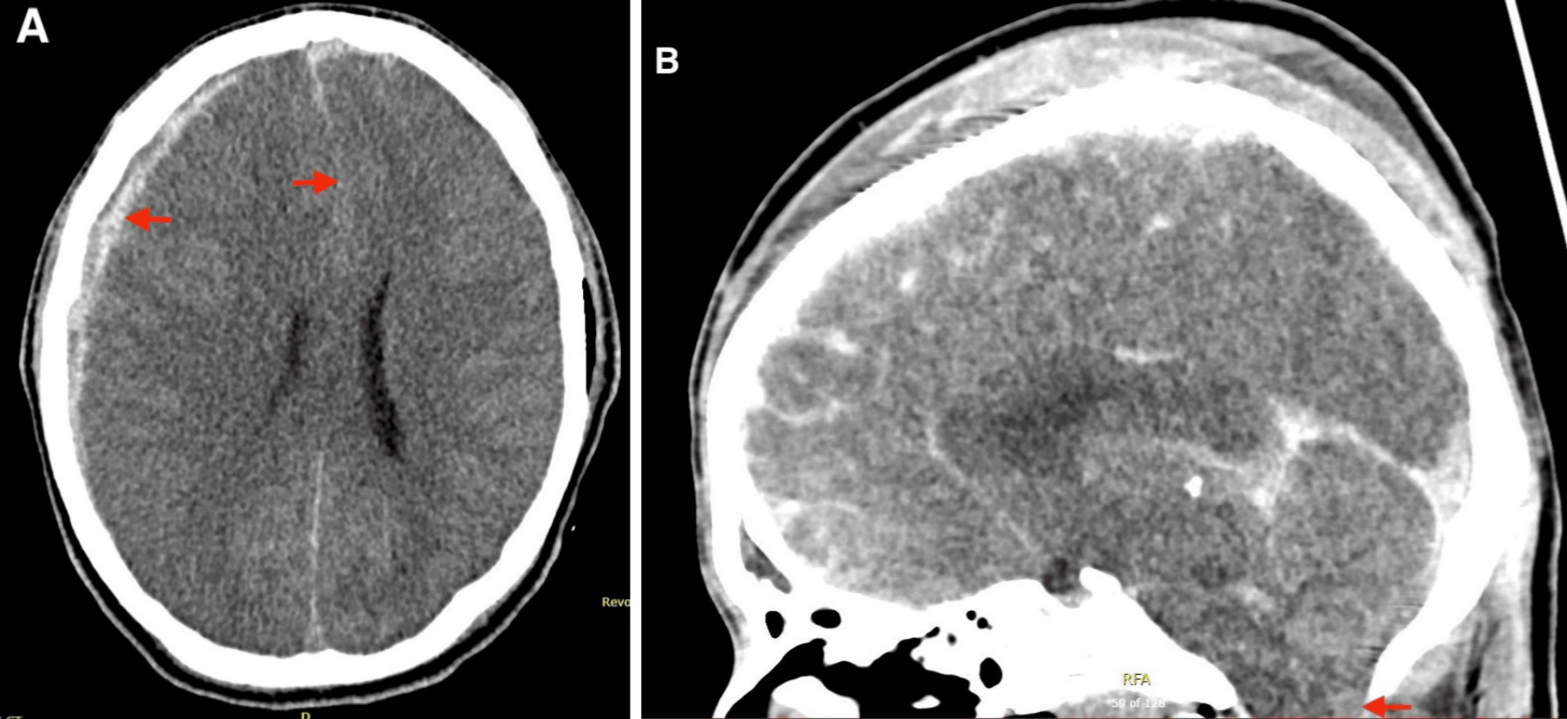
A) brain CT of the presenting patient showed a right-side subdural hematoma with midline shift; B) sagittal image demonstrates effacement of CSF space in the foramen magnum and tonsillar herniation CT - computed tomography; CSF - cerebrospinal fluid
